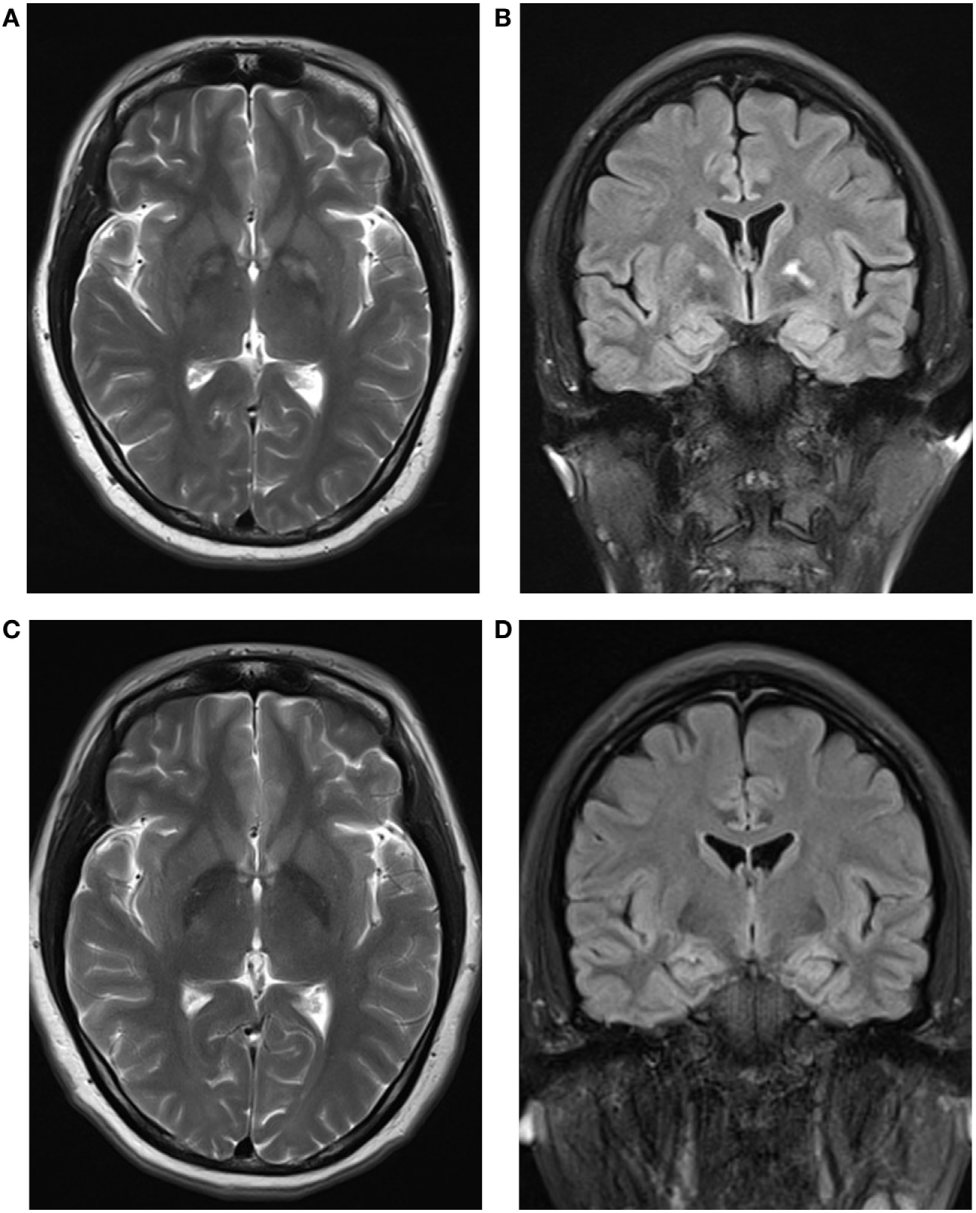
(A,C) Axial T2-weighted magnetic resonance imaging (MRI) slices showing symmetrical hyperintensity in the globus pallidus at 17 days (A) and 3.5 months (C) after poisoning, demonstrating a reduction in globus pallidus hyperintensity. (B,D) Coronal fluid-attenuated inversion recovery MRI slices at 17 days (B) showing asymmetry of the hippocampus larger on the left side and improved symmetry at 3.5 months (D) after poisoning.
